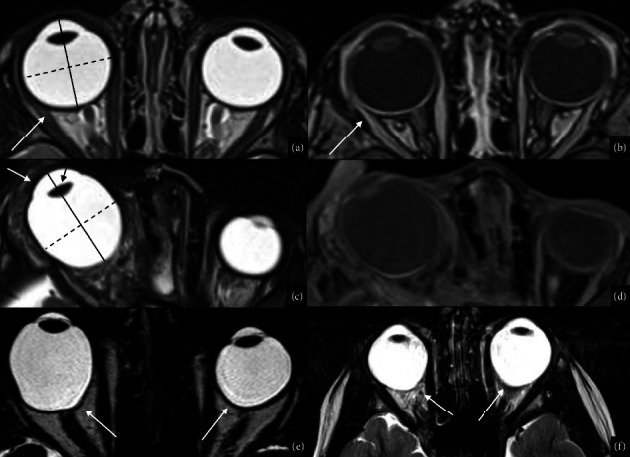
Macrophthalmia (macrophthalmus, buphthalmos, and axial myopia) and Staphyloma. MRI T2 FS WI (a) and postcontrast T1 FS WI (b) of a four-year-old boy with right macrophthalmus (white arrows in (a, b)). Macrophthalmus is characterized by a homogeneous increase in size (anteroposterior, continuous black line in (a); and transverse diameters, dotted black line in (a)) of the ocular globe. MRI T2 FS WI (c) and postcontrast T1 FS WI (d) of a one-year-old boy with right buphthalmos, secondary to congenital glaucoma. Buphthalmos is characterized by a prevalent enlargement of the anteroposterior diameter (continuous black line in (c)) compared to the transverse diameter (dotted black line in (c)). Increased diameter of the cornea and flattening of the ventral surface of the lens are also appreciable (white and black arrows, respectively). Axial DRIVE (e) of a ten-year-old girl affected by axial myopia in the left eye and bilateral staphyloma. Axial myopia is characterized by an increased size of the ocular globe and in particular an increased anteroposterior depth of the posterior segment of the eye. It may frequently be complicated by staphyloma, consisting of a posterior uveoscleral bulge, frequently appreciable on the nasal side of the optic disc (white arrows in (e)). MRI T2WI (f) of a six-year-old boy affected with bilateral staphyloma, which consists of posterior uveoscleral bulges, appreciable on the nasal side of the optic disc (white arrows in (f)).
